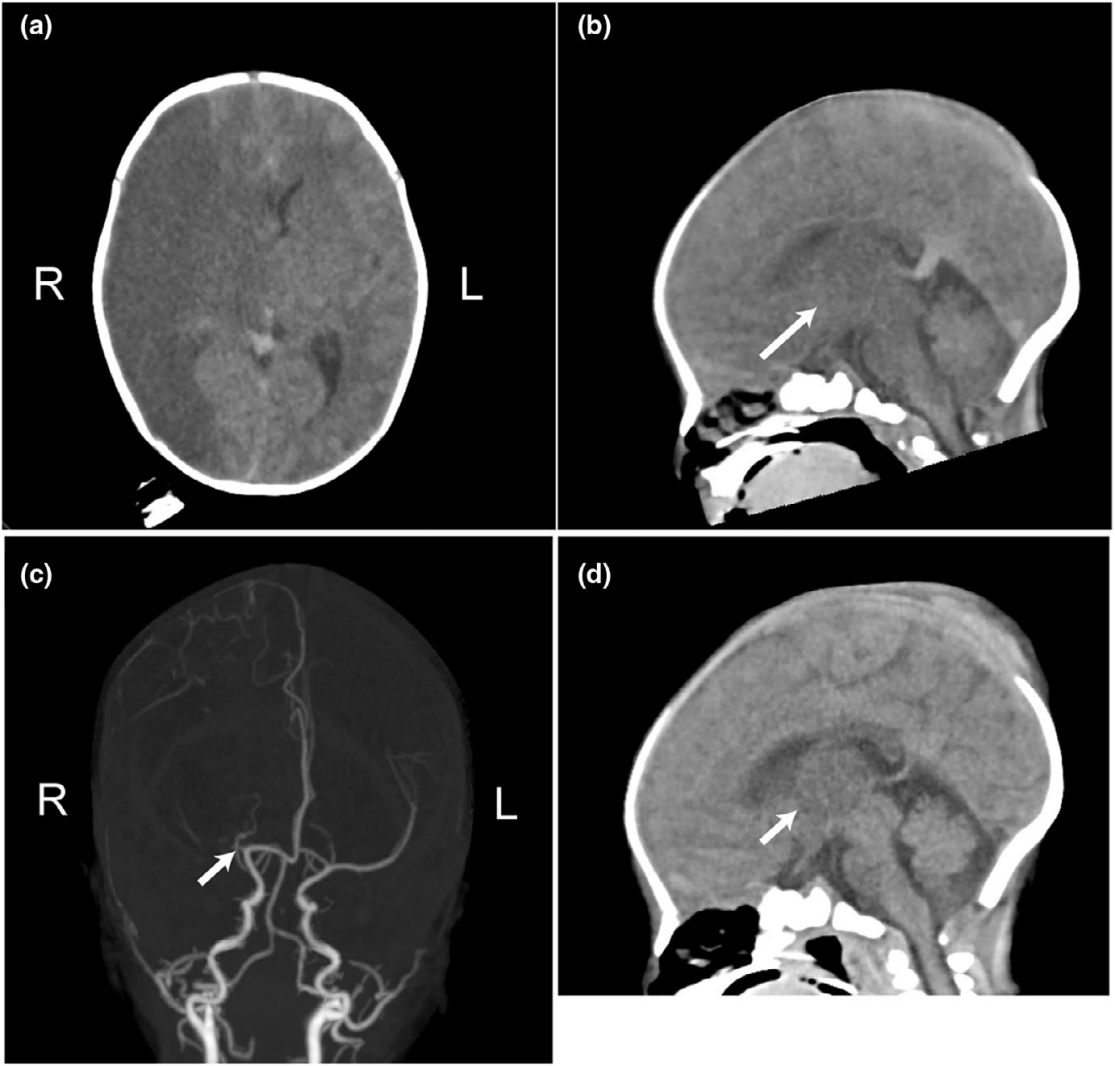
(a, b) Computed tomography (CT) at diagnosis: Diffuse homogeneous hypodensity of the right hemisphere and multi‐focal edema of the contralateral hemisphere with right to left midline shift (a) and hypodensity of the left thalamus (b, an arrow). (c) CT angiography: The right middle cerebral artery occlusion (an arrow). (d) CT at POD 1: Marked improvement of ischemia/edema of the thalamus (an arrow).
